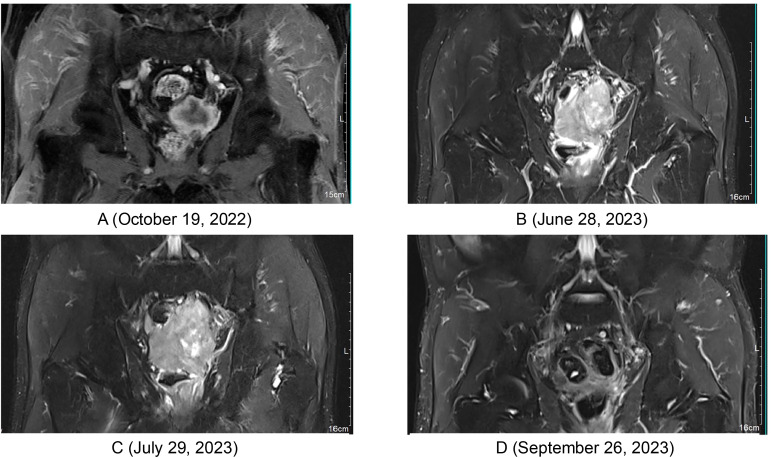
Serial pelvic MRI enhanced scans showing disease course. (A) The pelvic MRI enhanced scan showed a mass shadow on the left side of the rectal region, indicating metastasis. (B) The pelvic MRI enhanced scan revealed an increase in the size of the mass shadow on the left side of the rectum, indicating disease progression. (C) The pelvic MRI enhanced scan indicated stable disease after two cycles of treatment with eribulin and sintilimab. (D) The pelvic MRI enhanced scan indicated a significant reduction in tumor size compared to the previous scan after receiving the combination of high-intensity focused ultrasound, combined with eribulin and sintilimab.
